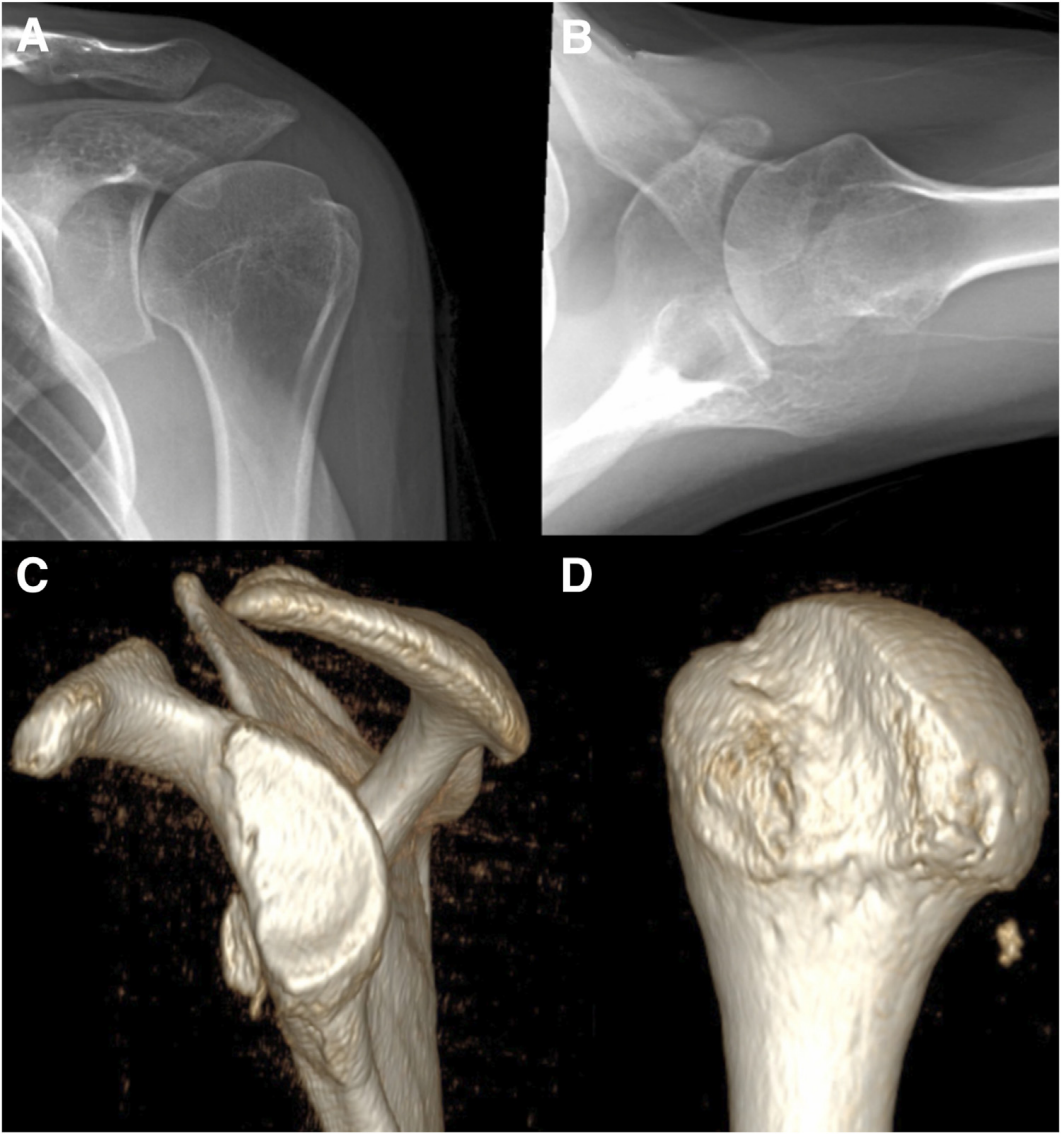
Preoperative radiographs and three-dimensional (3D) computed tomography (CT) reconstruction of the left shoulder. The large Hill-Sachs defect, as well as the notable glenoid bone loss and anterior subluxation of the humeral head can be appreciated. (A) Left shoulder anterior-posterior radiograph. (B) Left shoulder axillary lateral radiograph. (C) Left glenoid 3D CT reconstruction showing magnitude of glenoid bone loss. (D) Left humeral head 3D CT reconstruction showing size of Hill-Sachs lesion.
